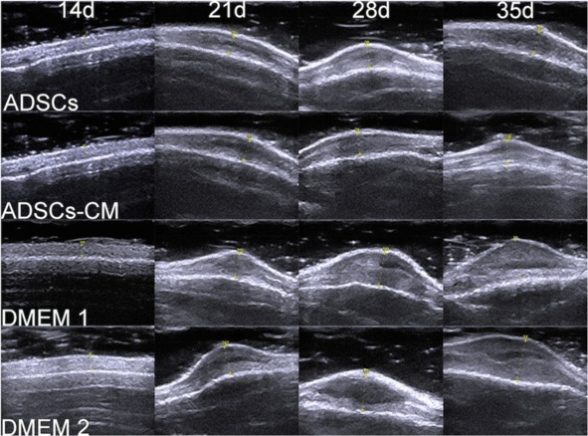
Ultrasonography of the wounds. Ultrasonography shows a gradually growing thickness of the scars in the internal control sides (DMEM 1 and DMEM 2) but almost no change in the ADSC- and ADSCs-CM-treated scars. *ADSC* adipose-derived stem cell, *ADSCs-CM* conditioned medium of the adipose-derived stem cells, *DMEM* Dulbecco’s modified Eagle’s medium
